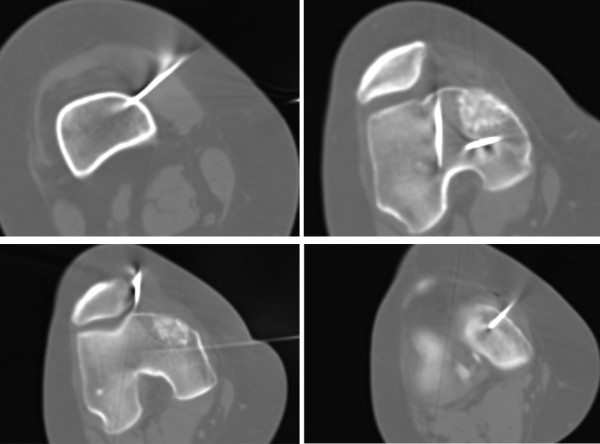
CT scan showing the position of K-wires to define surgical margins. In the upper left square the K-wire is inserted proximally to the lesion; in the upper right square two K-wires delimit the lesion laterally in the proximal part; in the lower left square two K-wires delimit the lesion laterally in the distal part; in the lower right the k-wire is inserted distally to the lesion.
